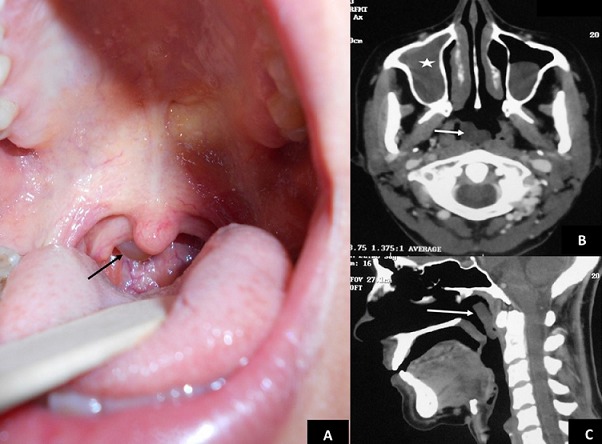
(A) clinical examination showing a pale, spherical mass hanging in the oropharynx (arrow); (B) axial computed tomography showed the complete opacification of the right maxillary sinus (star) and a polypoid mass of the nasopharynx (arrow); (C) sagittal computed tomography showed a polypoid mass of the nasopharynx (arrow)
